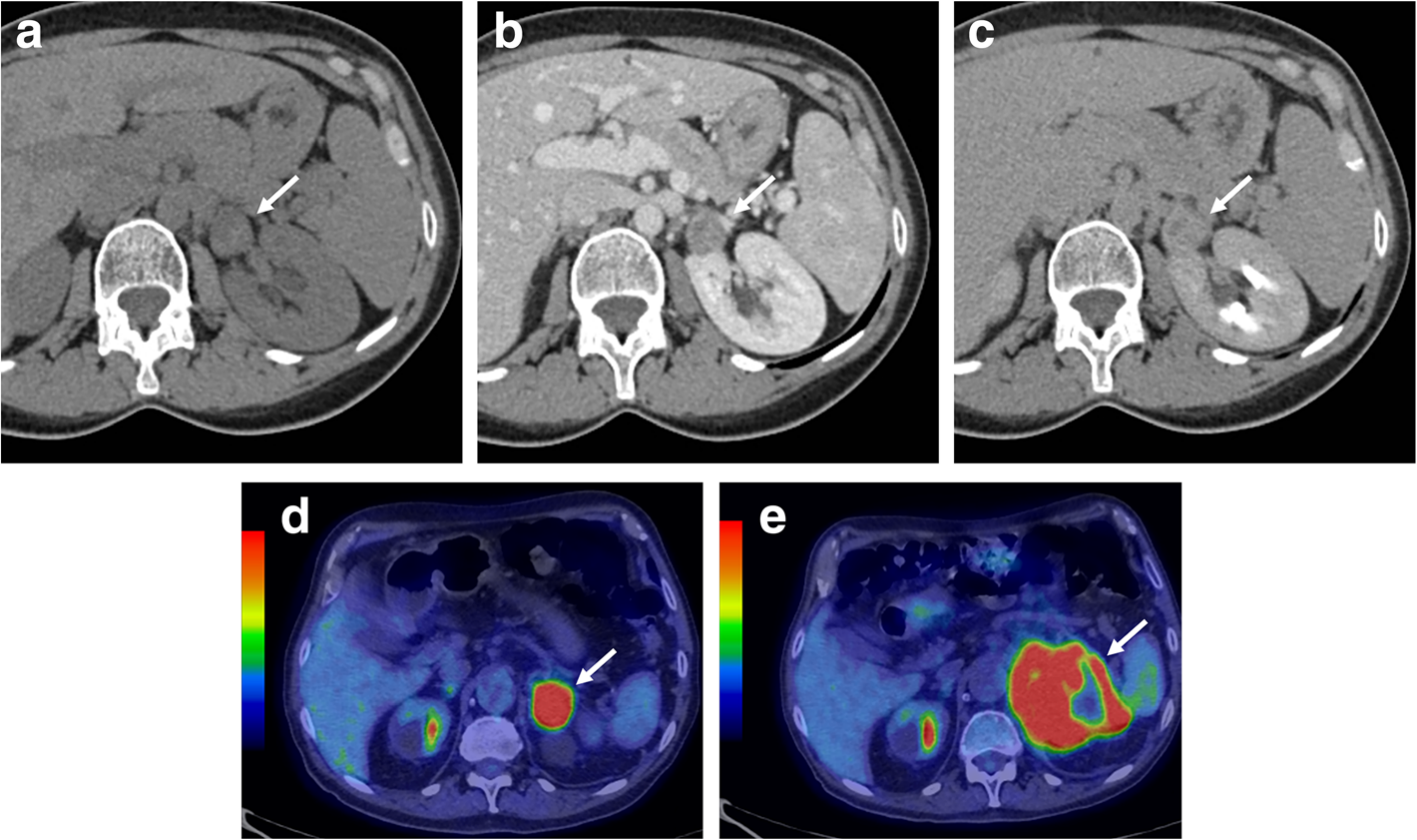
Left adrenal metastasis in a 62-year-old woman with lung cancer. Unenhanced CT (a), portal (b), and late phase (c) images show a left adrenal mass (arrow) with attenuation value of 26 HU on unenhanced CT (a) and “absolute percentage washout” of 15%. Staging ^18^F-FDG PET/CT (d) performed 3 months later revealed a left adrenal lesion (arrow) with high metabolic activity (SUV_max_ 14 g/mL) and increased in size in comparison with CT images. After chemotherapy, restaging ^18^F-FDG-PET/CT (e) showed morpho-functional disease progression of the adrenal lesion (arrow) with signs of central necrosis (SUV_max_ 19 g/mL with central necrotic area of absence of ^18^F-FDG uptake). *Images of*
